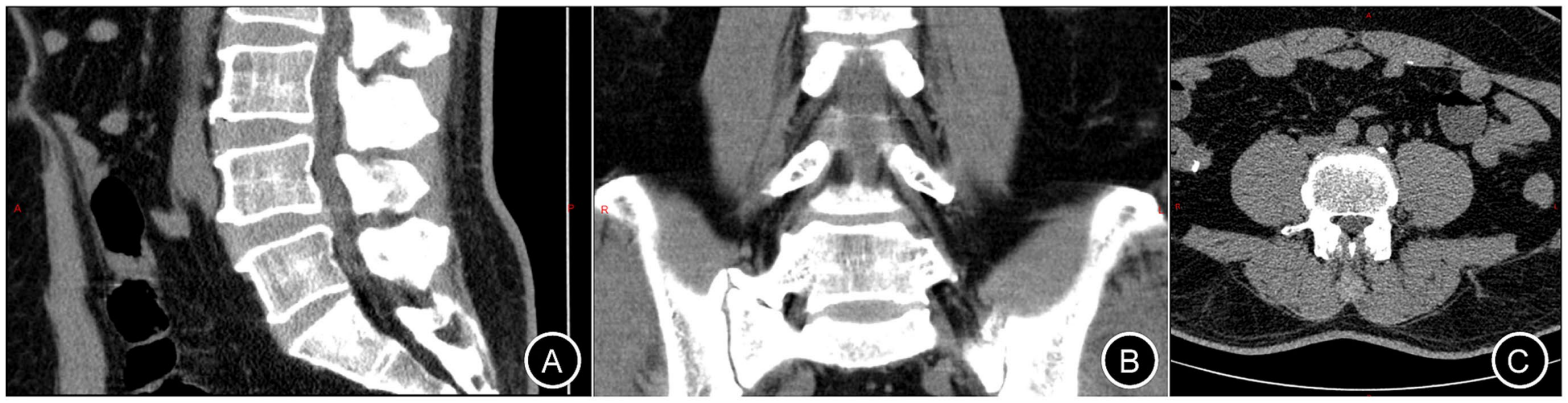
Lumbar intervertebral disk CT. Panels (A–C) correspond to the sagittal, coronal, and axial planes, respectively.
